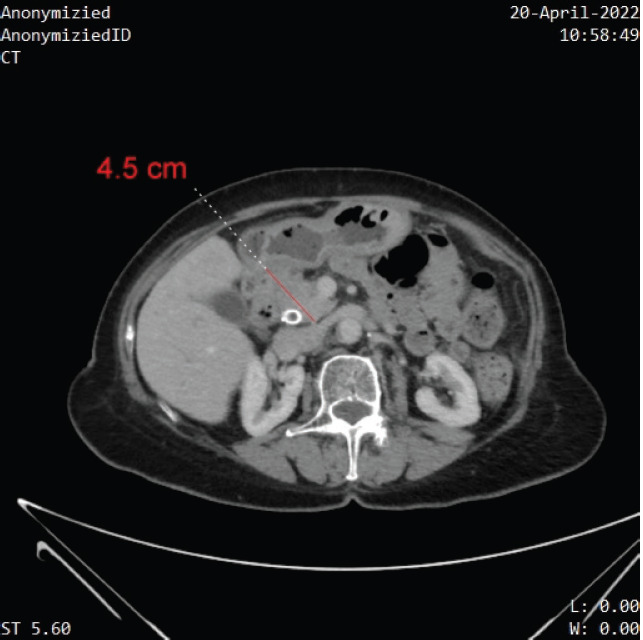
Contrast-enhanced CT demonstrating the stability of the mass at the head of the pancreas without further progression, or distant metastases.
